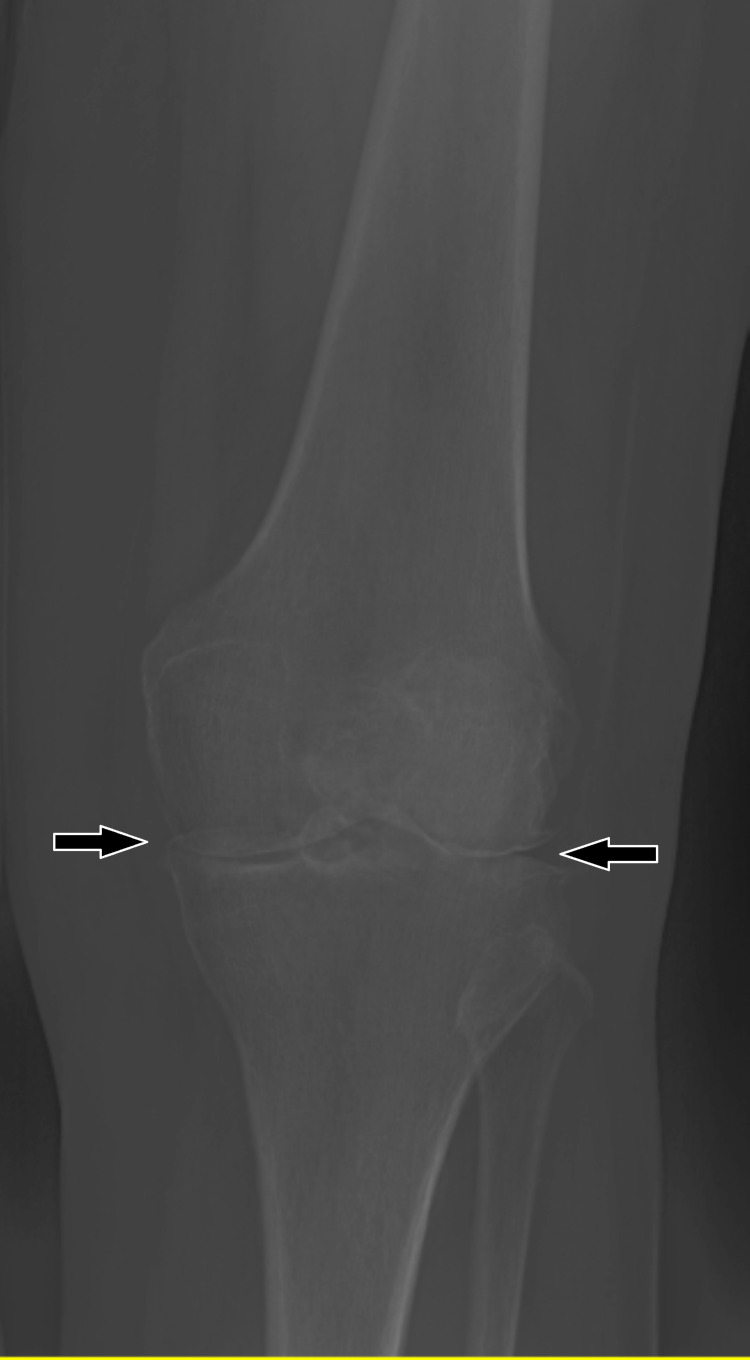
Left knee X-ray showing medial and lateral joint space narrowing consistent with osteoarthritis (black arrows).
